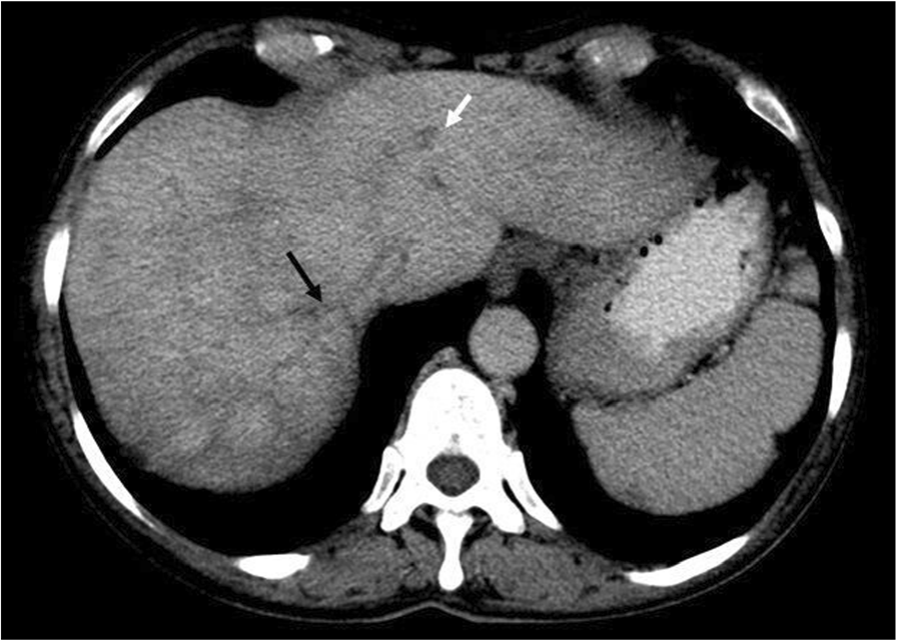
Portovenous phase axial CT demonstrating Budd-Chiari syndrome in a patient with myelofibrosis. The middle hepatic vein (black arrow) and left hepatic vein (white arrow) are occluded and non-enhancing, and the inferior vena cava (not shown) is non-opacifying and slit-like. Note the early ‘nutmeg liver’ appearance in segment 7 which is a typical feature of Budd-Chiari syndrome
